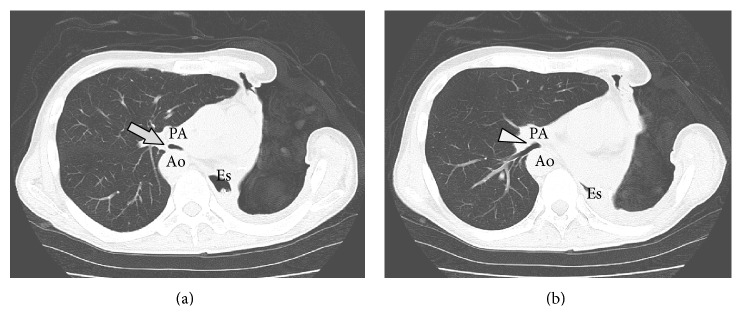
Computed tomography taken two weeks after mediastinal repositioning. Complete adhesiolysis of the left thoracic cavity was performed and a thoracostoma was created. Piles of gauze were inserted to maintain the proper mediastinum position. The compression of the bronchus was released, and the lumens of the bronchus intermedius ((a) arrow) and right lower bronchus ((b) arrowhead) were sufficiently patent. Ao, right descending aorta; PA, right pulmonary artery; Es, esophagus.
